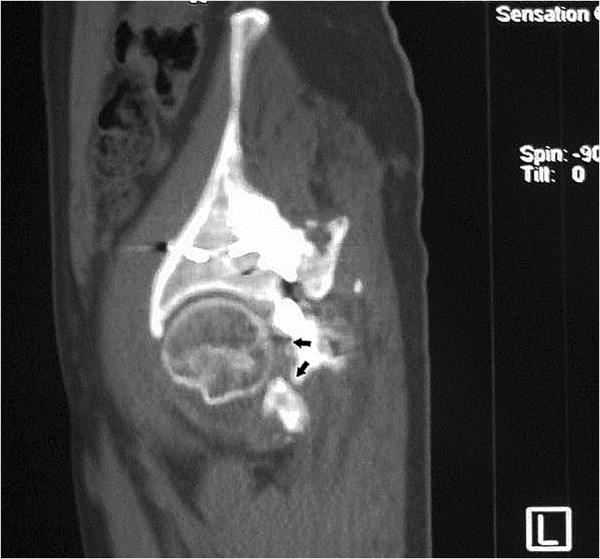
Coronal CT scan shows incongruent reduction of the hip joint and mal-union in the posterior wall and column of the acetabulum (arrows). However, more than 50% of joint space was maintained and no evidence of collapse or necrosis in femoral head was revealed.
